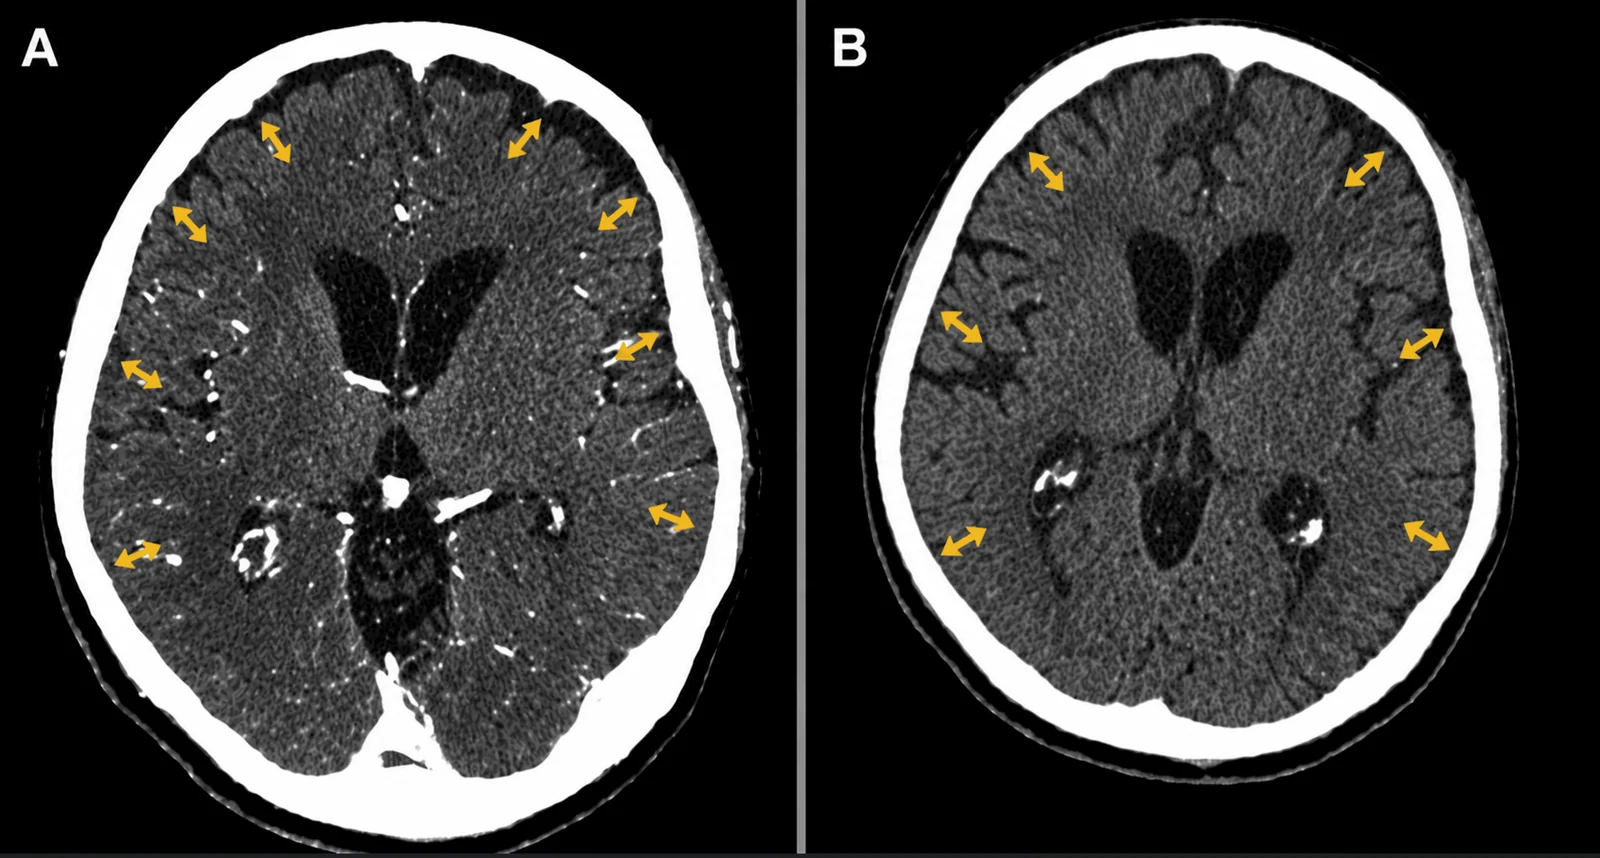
Initial contrast-enhanced brain CT demonstrating diffuse cerebral cortical atrophy. In panels A and B, the orange arrows indicate prominence and widening of the cortical sulci, consistent with generalized cortical volume loss. No acute focal intracranial lesion is identified.
